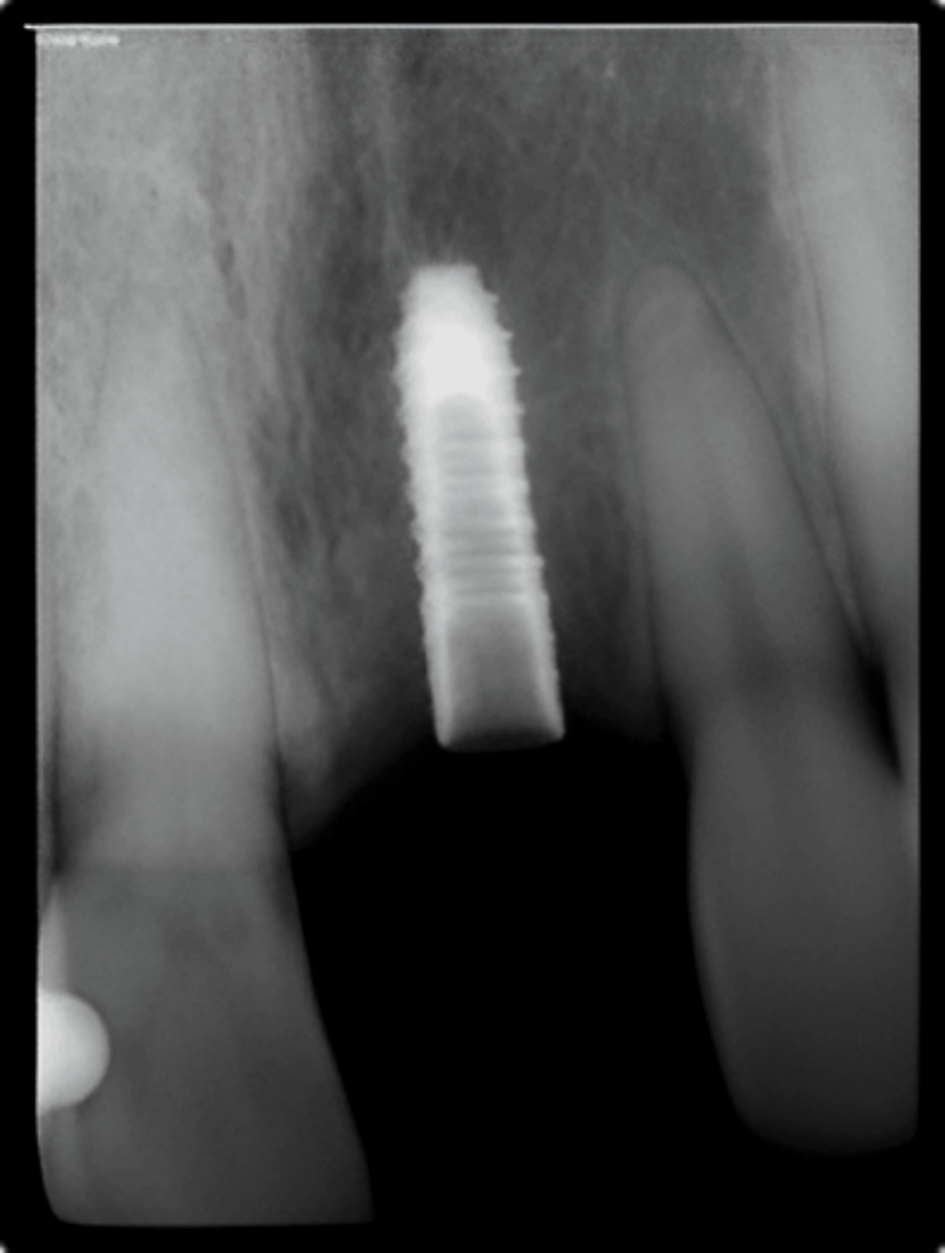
Case 3: Immediate postoperative IOPA depicting bone condensation with respect to 21 IOPA: Intra-oral periapical radiograph.
